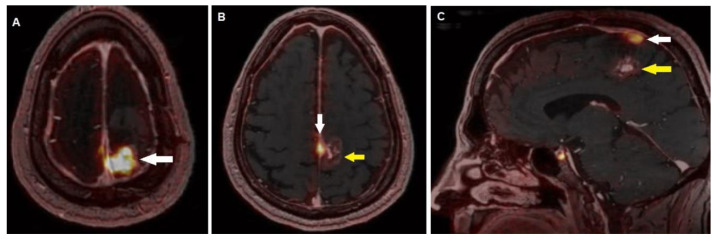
A 60-year-old male patient, previously submitted to surgery for a left parietal WHO grade III meningioma, then relapsed and treated with Gamma Knife. A 6-month post RT follow-up ^68^Ga-DOTATOC PET and contrast-enhanced T1-weighted co-registered MRI axials revealed increased tracer incorporation within the left parietal meningioma (A, white arrow), also detecting a focus of additional uptake in the *falx cerebri* (B, white arrow) adjacent to an area of “soap-bubble” contrast-enhancement of the brain parenchyma (B, yellow arrow), not characterized by meaningful tracer uptake, interpreted as radionecrosis. Sagittal fused PET/MRI (C) also well depicted the residual meningioma in the parietal region (white arrow) and the radionecrosis (yellow arrow).
